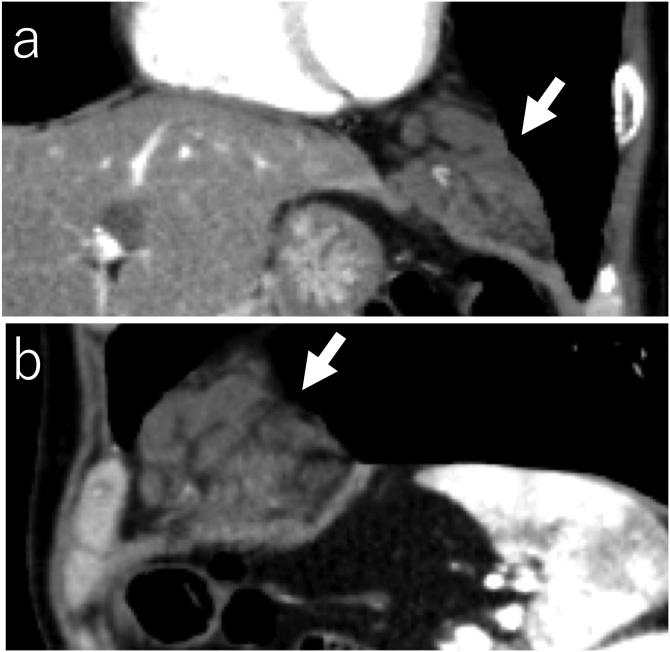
a) Chest contrast-enhanced computed tomography showing an irregular mass (arrows) with a maximum diameter of 55 mm in the anterior mediastinum, which extends along the left diaphragm (a: coronal plane, b: sagittal plane).
